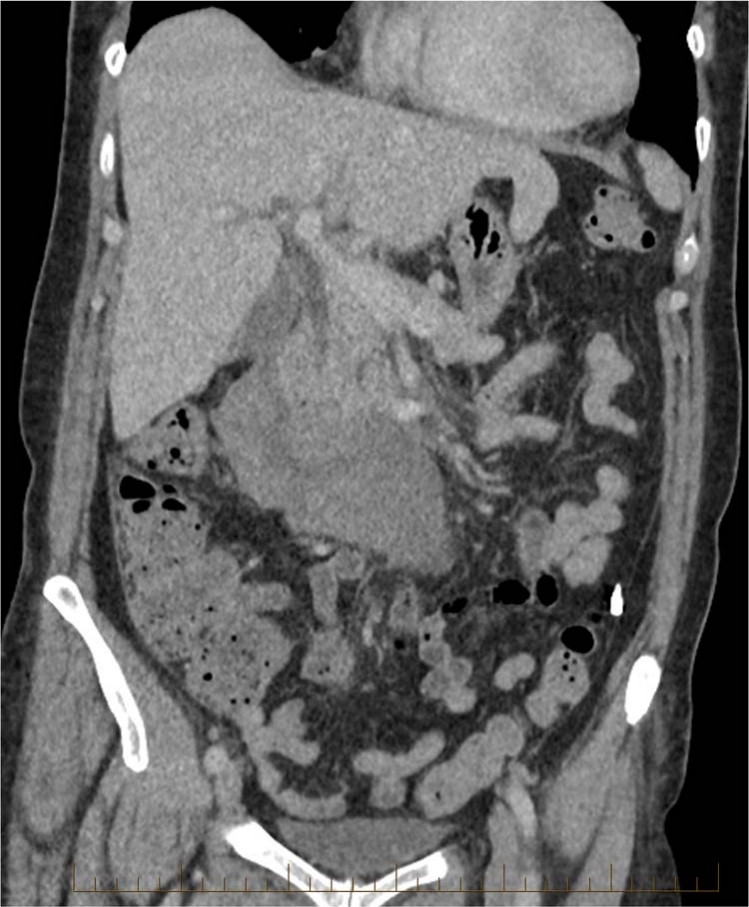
CT abdomen, coronal view. Slight interval decrease in size of previously identified periduodenal hematoma.
